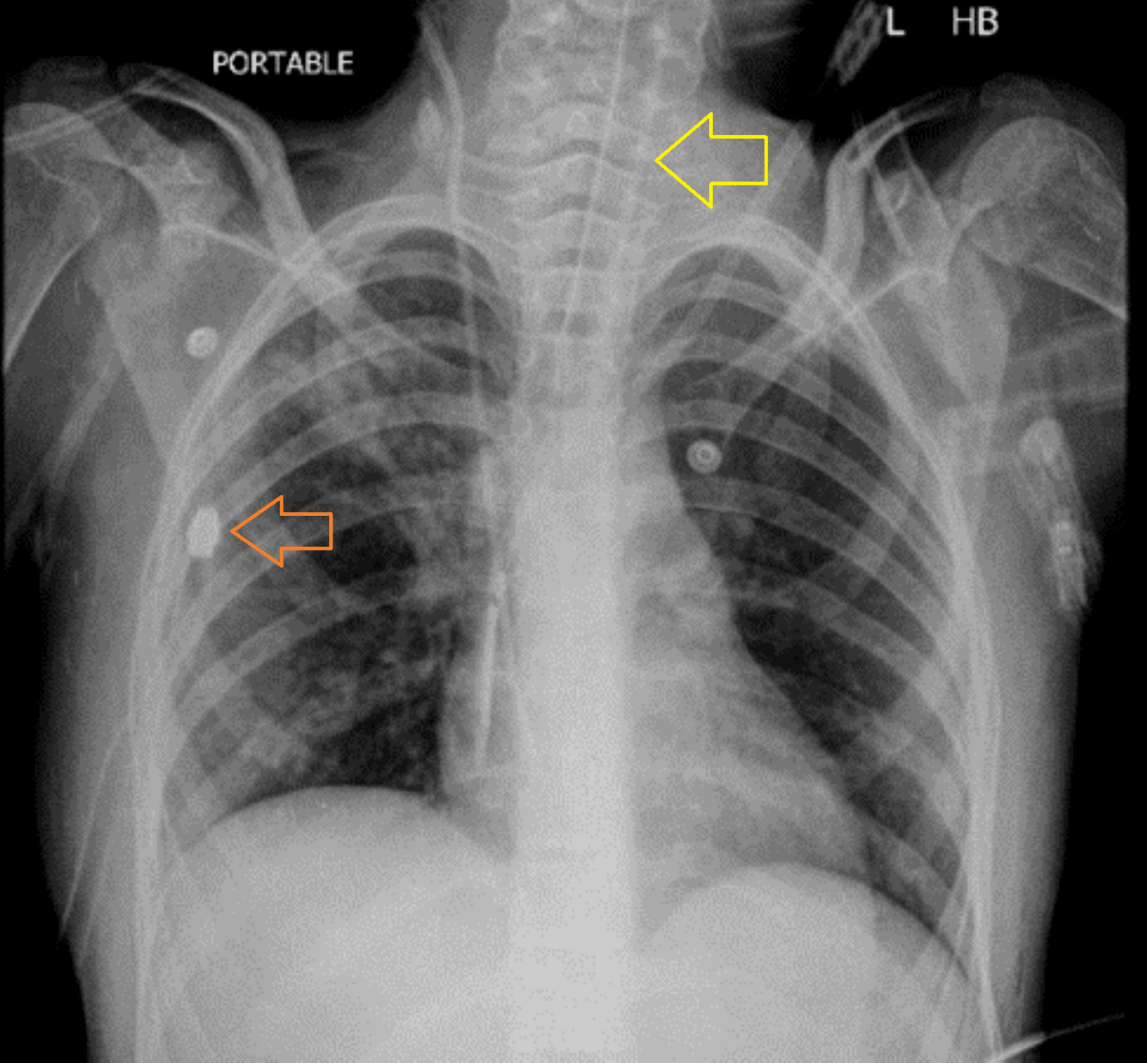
Chest X-ray on the morning of the eighth day of hospitalization with the chest tube (orange arrow) and endotracheal tube (yellow arrow) in place
